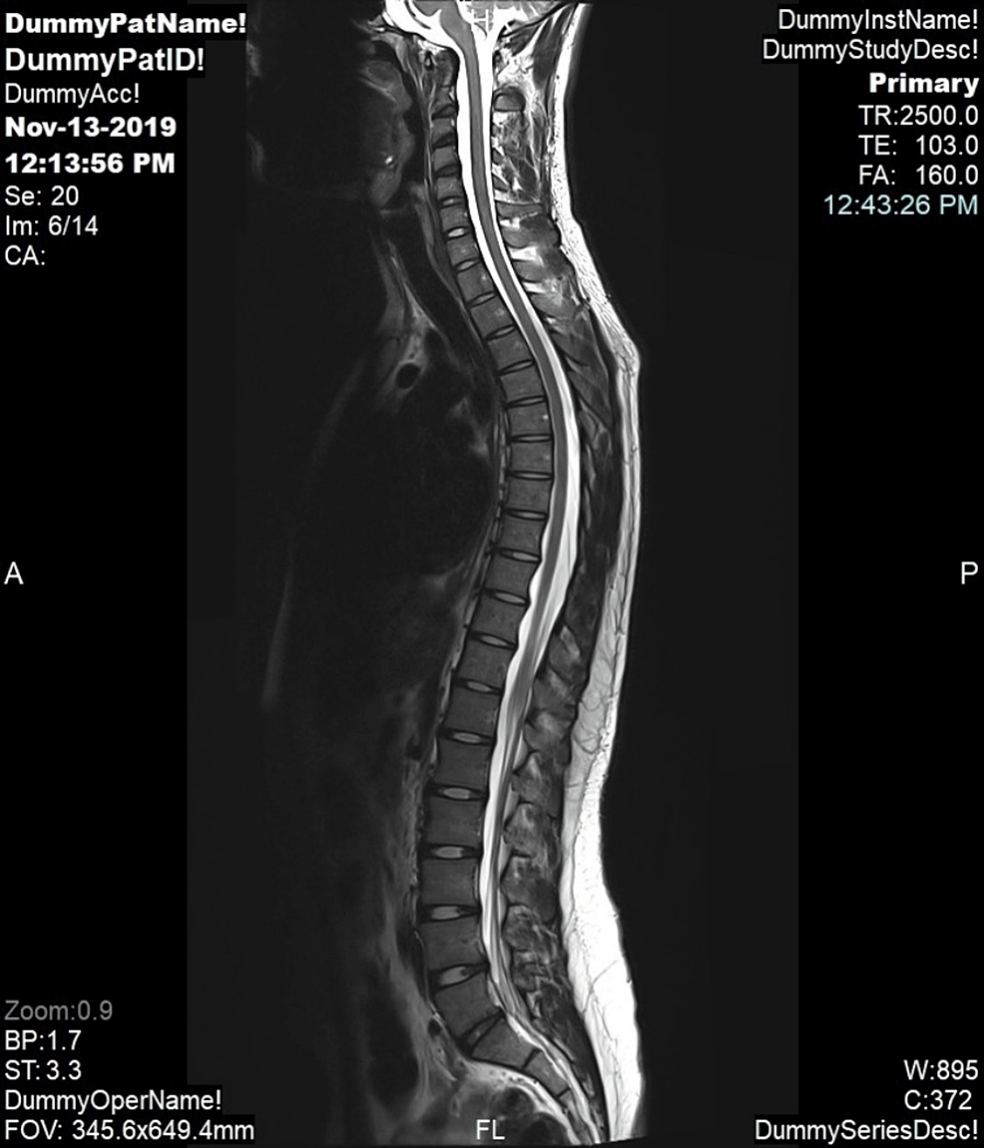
Magnetic resonance imaging of the thoracic spine with dural ectasia
